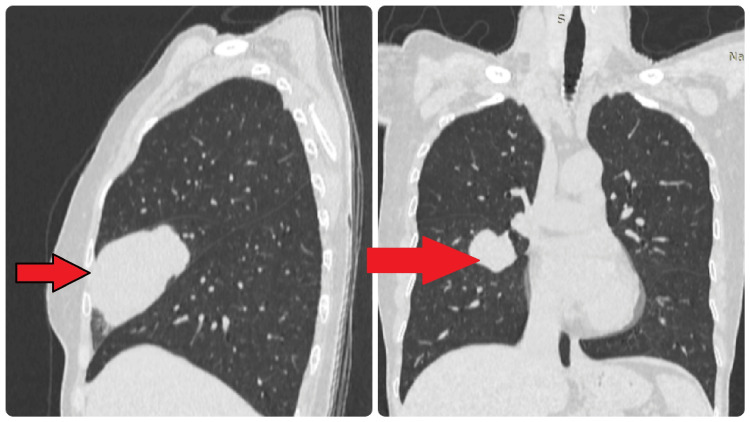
Contrast CT images indicate well-defined, isolated metastatic deposits in the right middle lobe (red arrows).
